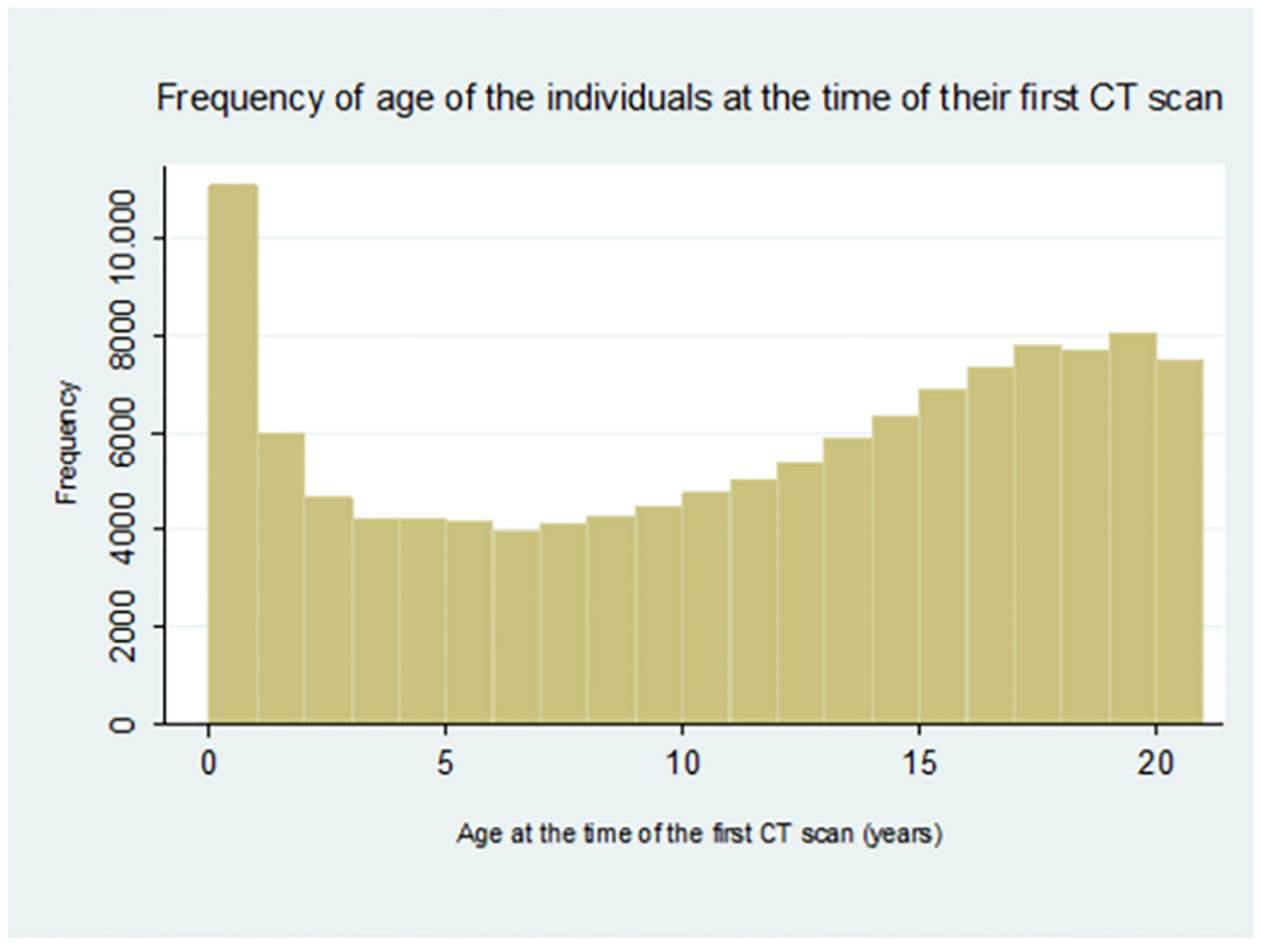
Frequency of age of the individuals at the time of their first CT scan.
